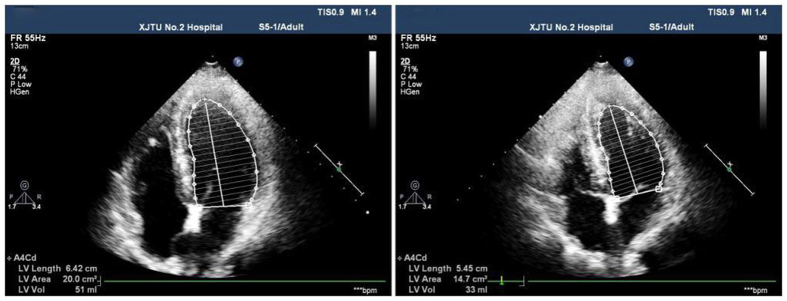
Measurement of the right atrial area in apical 4-chamber views at left ventricular end-systole at rest and during the Valsalva maneuver.
